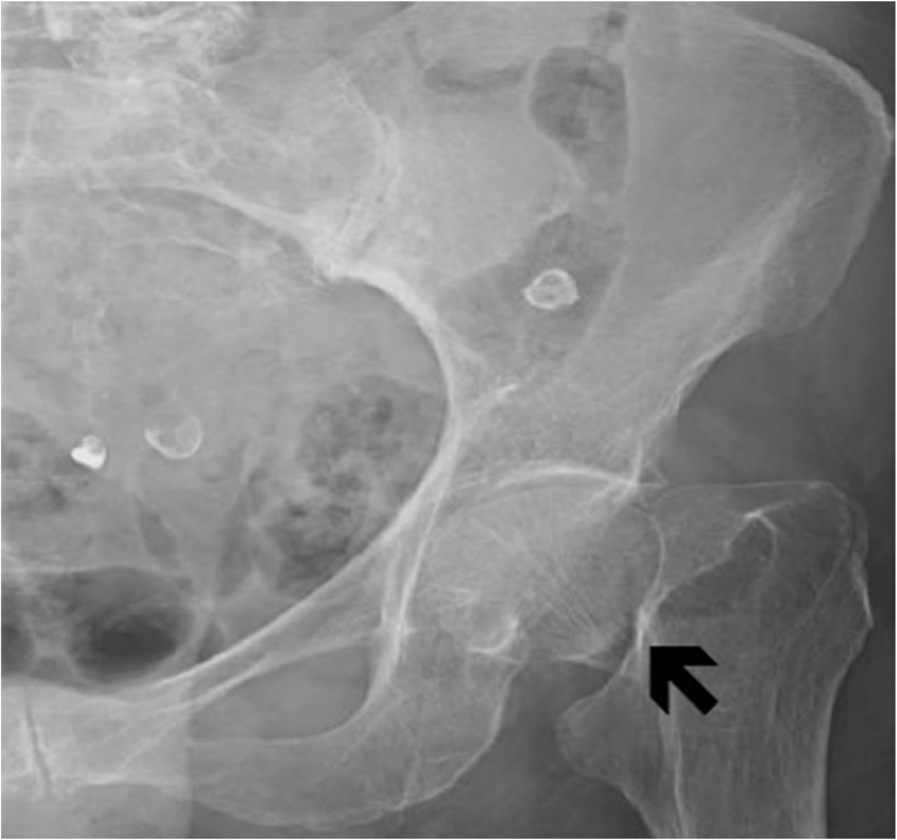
X-ray of the left hip and femur: a left subcapital femoral neck fracture (black arrow) can be seen.
